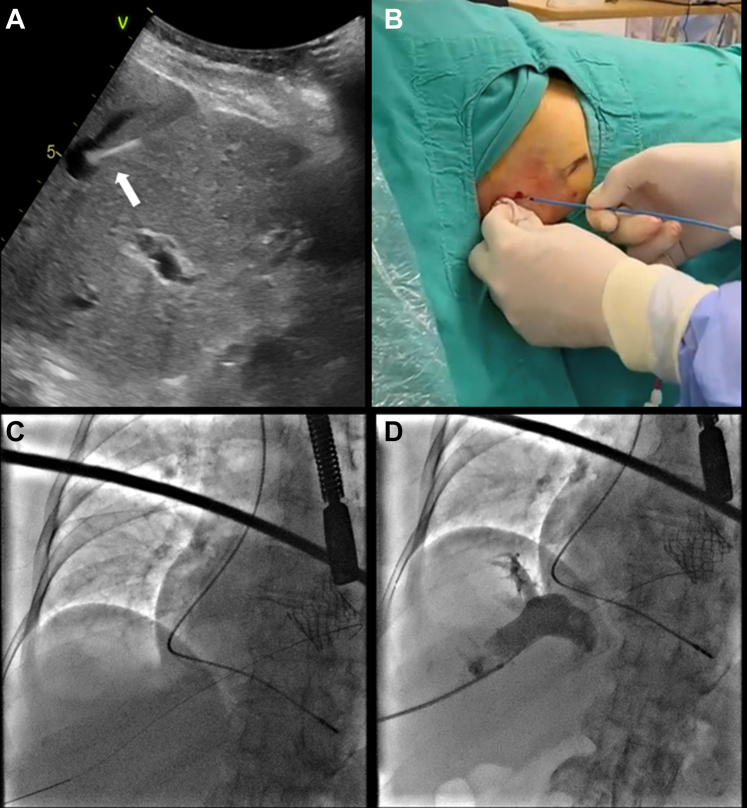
Suprahepatic Venous Access (A) Ultrasound-guided percutaneous puncture of SHV (white arrow) and (B) dilation with 4-F catheter. (C) Guidewire progression to SHV-RA junction. (D) Venogram confirming adequate access. RA = right atrium; SHV = suprahepatic vein.
